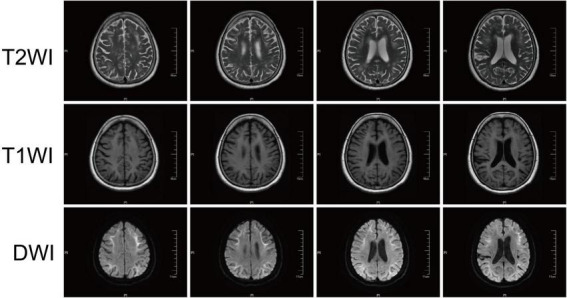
The typical brain magnetic resonance imaging (MRI) in patients with NIID. Curved or ribbon-like high signals along the corticomedullary junction, a strong clue for NIID, are found in the DWI sequence of head MRI of some patients with NIID.
